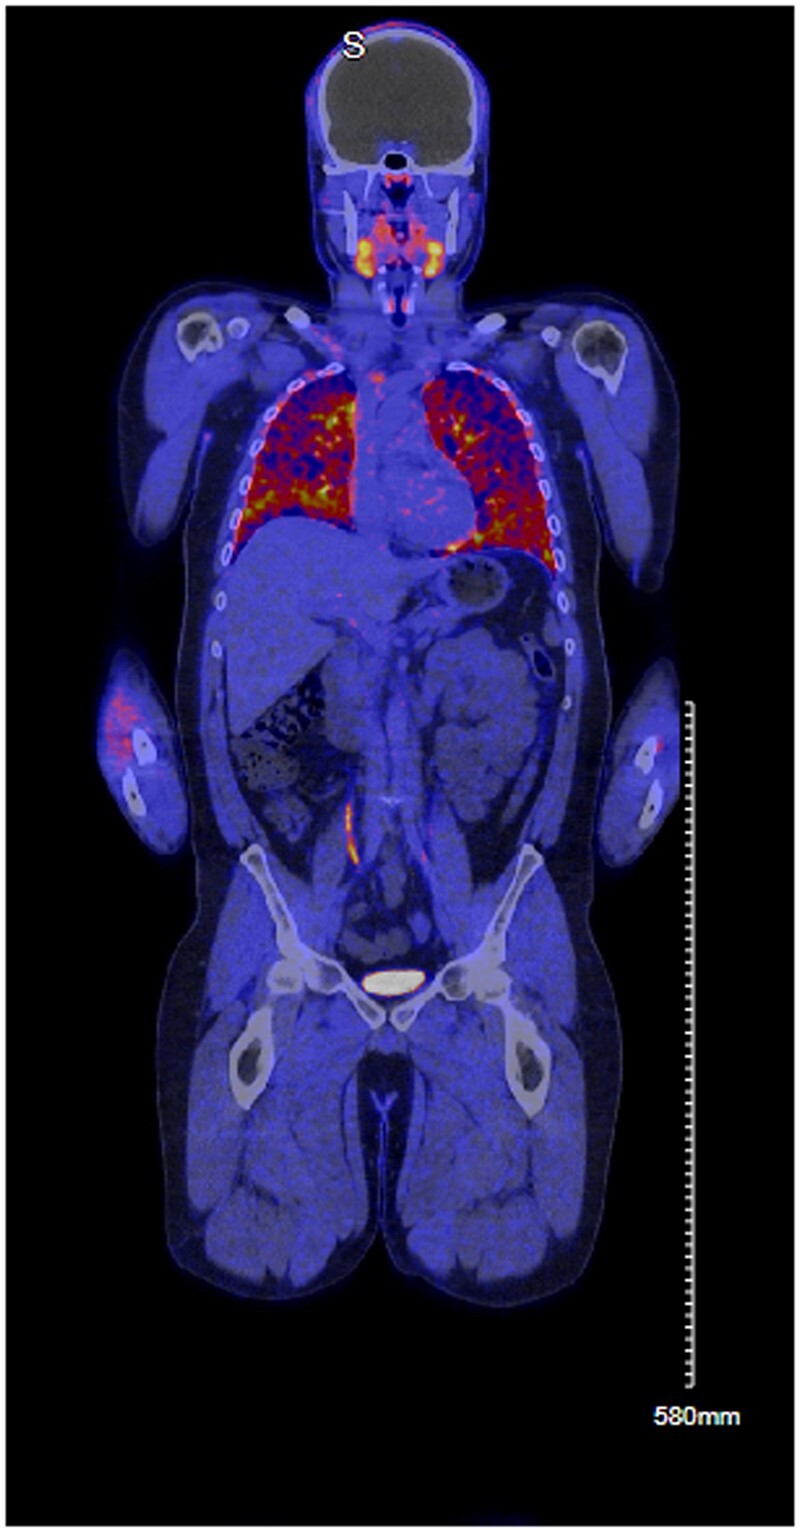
68Ga-FAPI-46-PET/CT demonstrated intensive FAPI uptake in the lung parenchyma (as depicted using the Hot Metal Colour Scale with blue areas signifying low uptake and orange and yellow areas signifying higher uptake).
